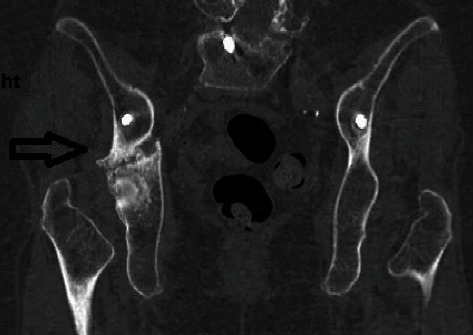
Frontal CT reconstruction image of the pelvis.
